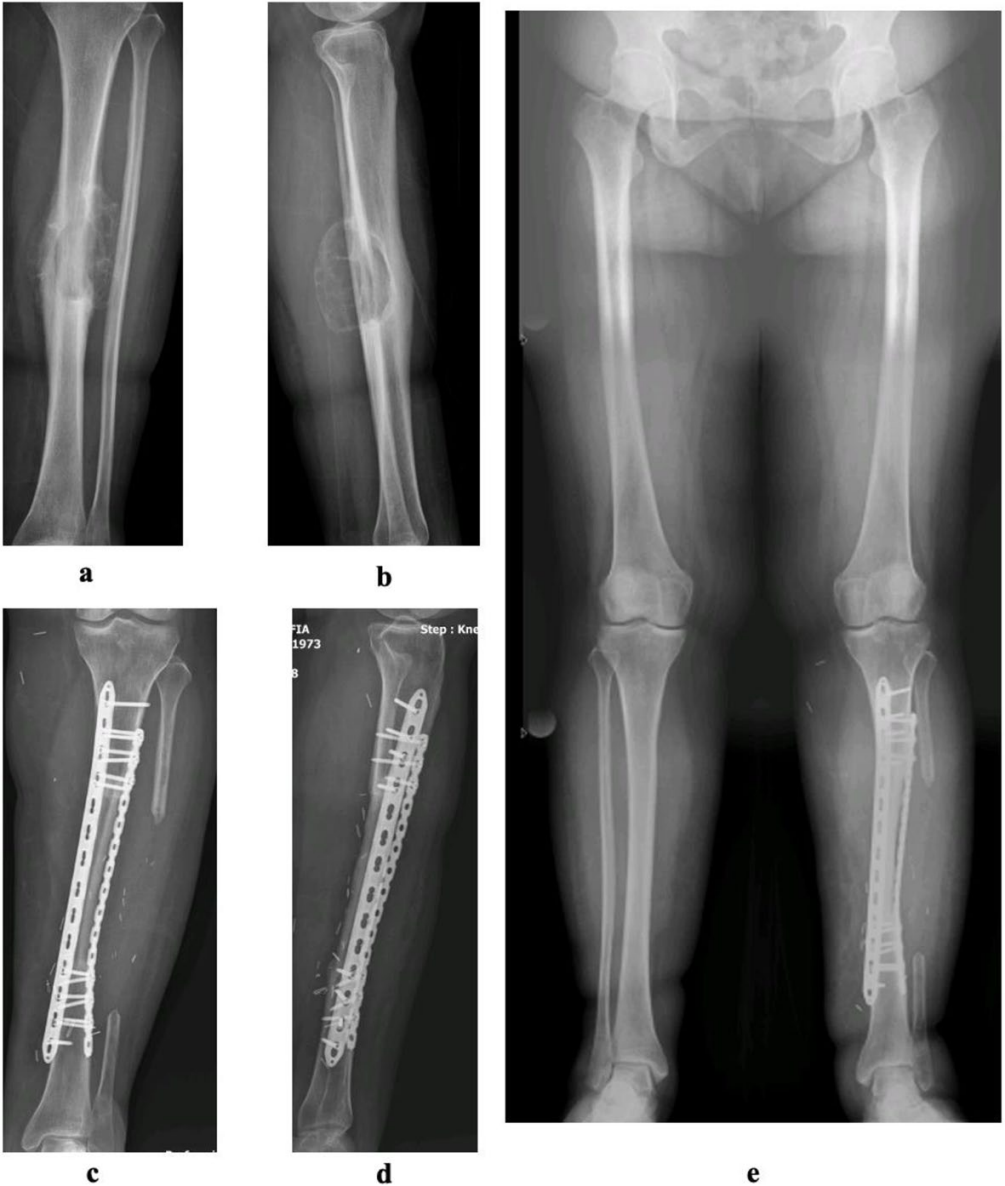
a Preoperative AP image of ABC in the diaphyseal tibial region. b Lateral plan view. c AP image of a patient who underwent reconstruction with vascularized fibula in the second postoperative year. d Postoperative lateral plan view. e Leg length radiography at eighth postoperative year
